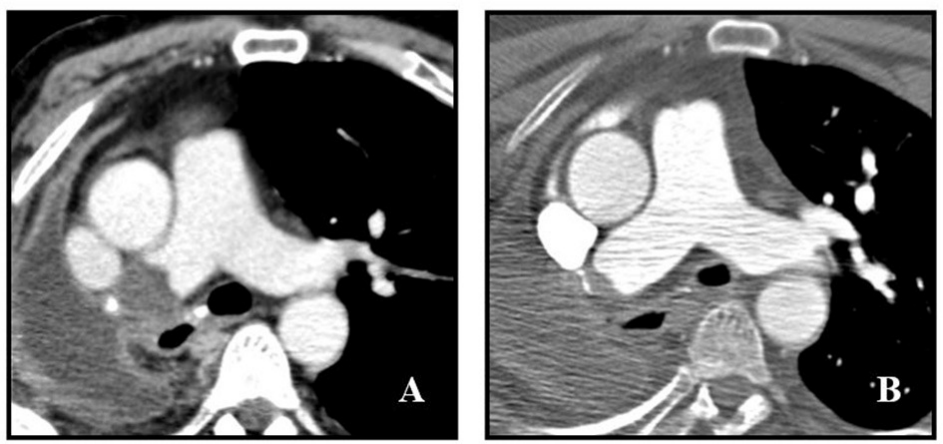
Post-pneumonectomy thrombosis of right pulmonary artery stump. (A) Initial contrast-enhanced CT scan shows a large endoluminal filling defect in the right pulmonary artery stump. (B) Follow up contrast-enhanced CT scan obtained 4 months after (A) shows marked reduction of thrombus size with evidence of a small residual intraluminal defect adjacent to surgical clips.
